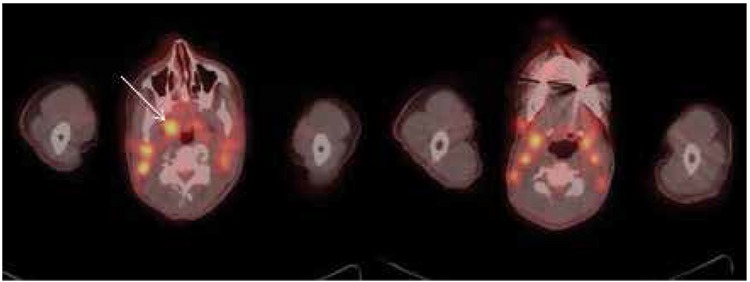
Increased fluorodeoxyglucose uptake in the deep cervical lymph nodes bilaterally, the largest and most avid node is noted in the right retropharyngeal region measuring 1.1x1.4 cm with SUVmax: 4.2 (arrow)
